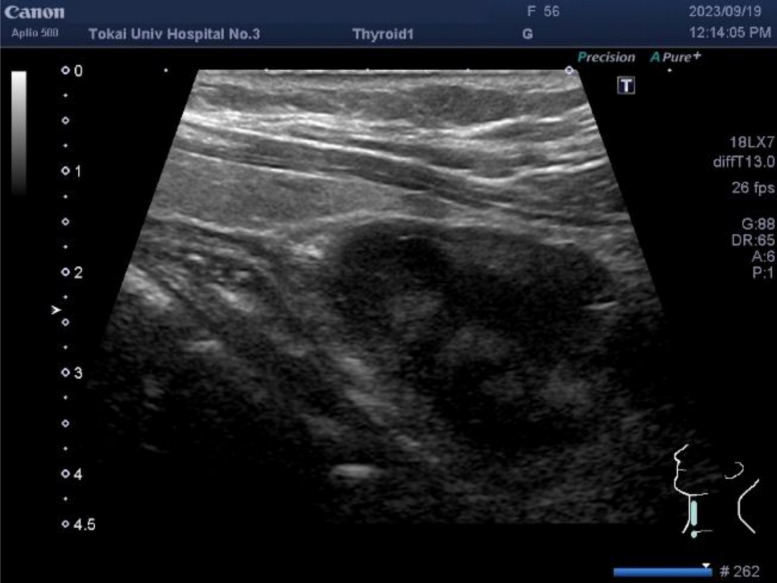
Cervical ultrasonography demonstrated a 37-mm well-defined mass located lateral to the lower pole of the thyroid gland. The lesion exhibited internal cystic degeneration
